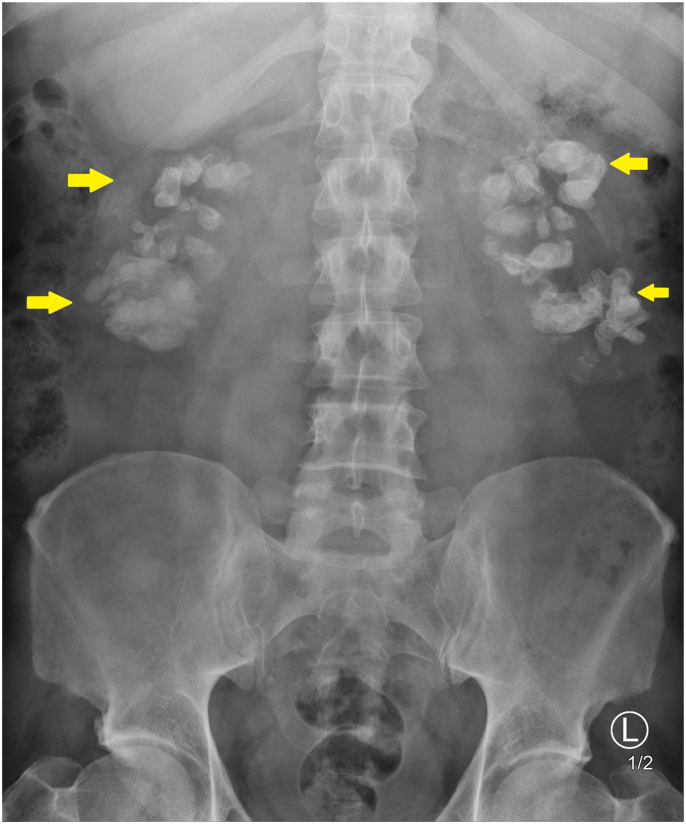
X-ray KUB of the abdomen. Significant bilateral calcifications are shown to be projected over each kidney.
